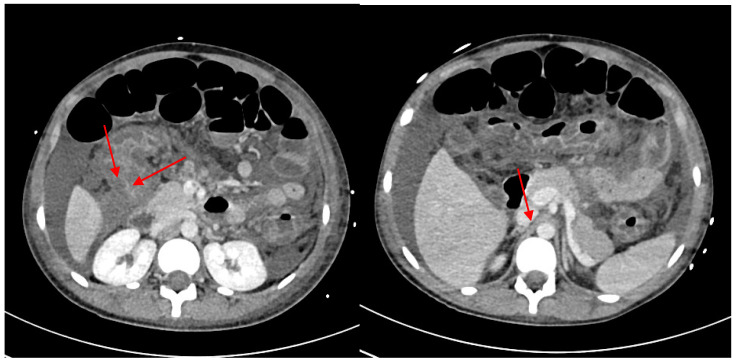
CT scan of superior mesenteric vein and splenic vein thrombosis.
